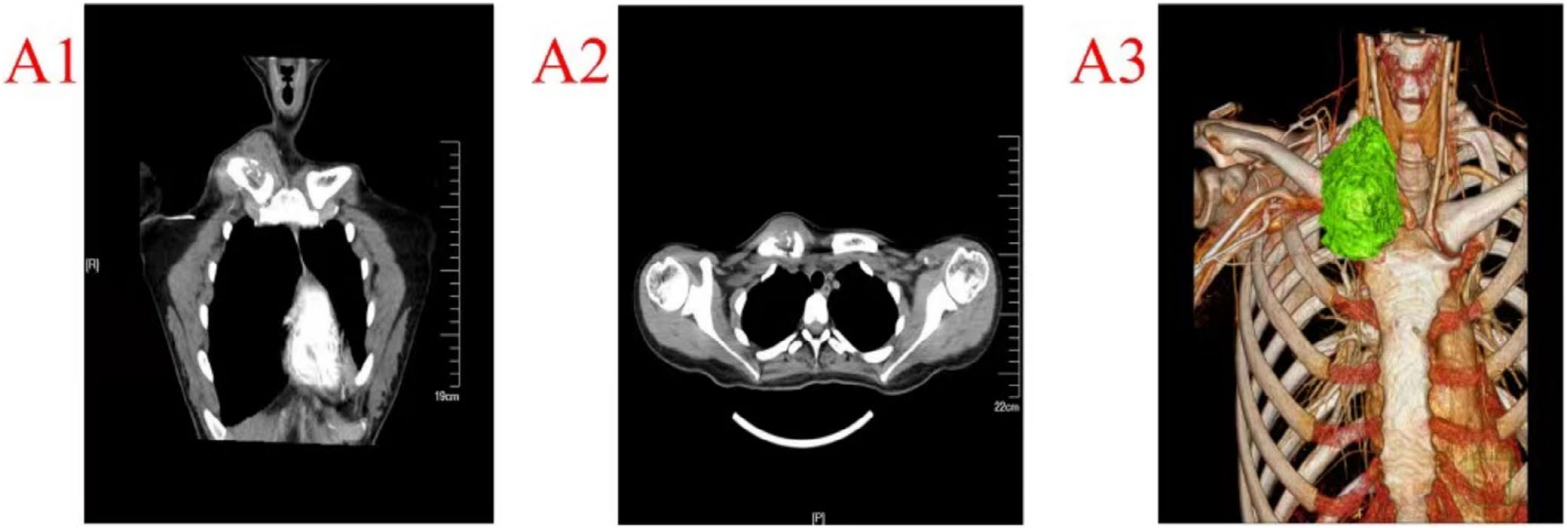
(A1–A3) Preoperative computed tomography angiography shows obvious tumor destruction of the medial end of the right clavicle and adjacent soft tissue erosion.
